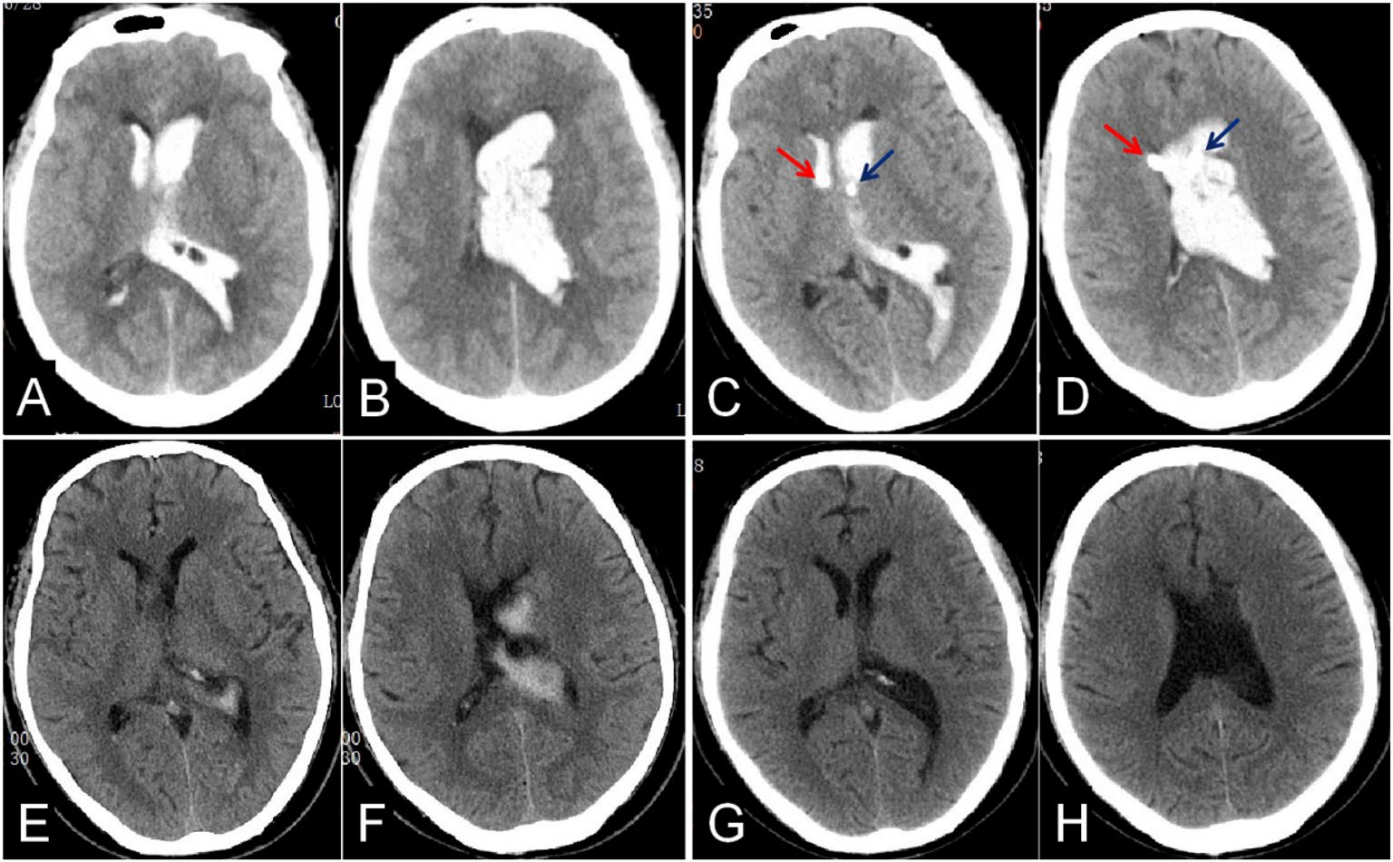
The optimal placement of EVD catheter was shown by the CT scan. IVH was shown at 3 h pre-operation (A,B); The locations of the EVD catheters were shown at 1 d post-operation with iUS-guided (the left catheter shown by the red arrow, the right catheter shown by the blue one, (C,D); There was about 80% IVH removal, and the catheter was removed at 2 weeks after EVD (E,F); And there was no hydrocephalus at 1 m follow-up (G,H).
